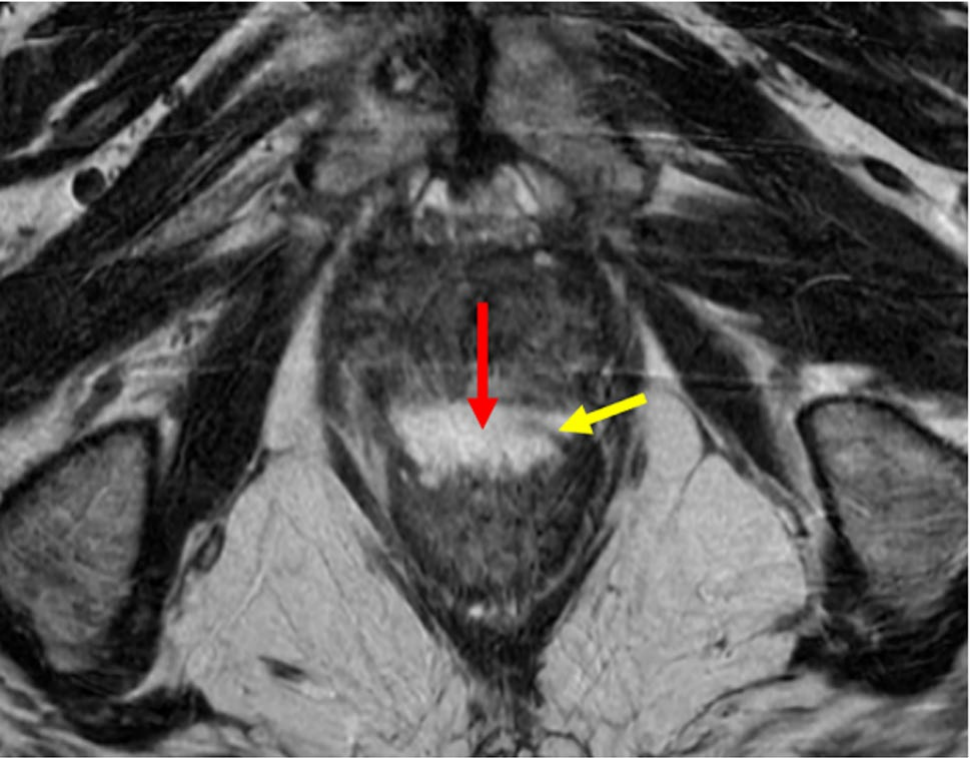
Routine post procedural axial T2 MRI shows infiltration of hydrogel material (red arrow) into the anterior rectal wall (yellow arrow) without disruption of the mucosa. Patient was asymptomatic during and after the procedure
